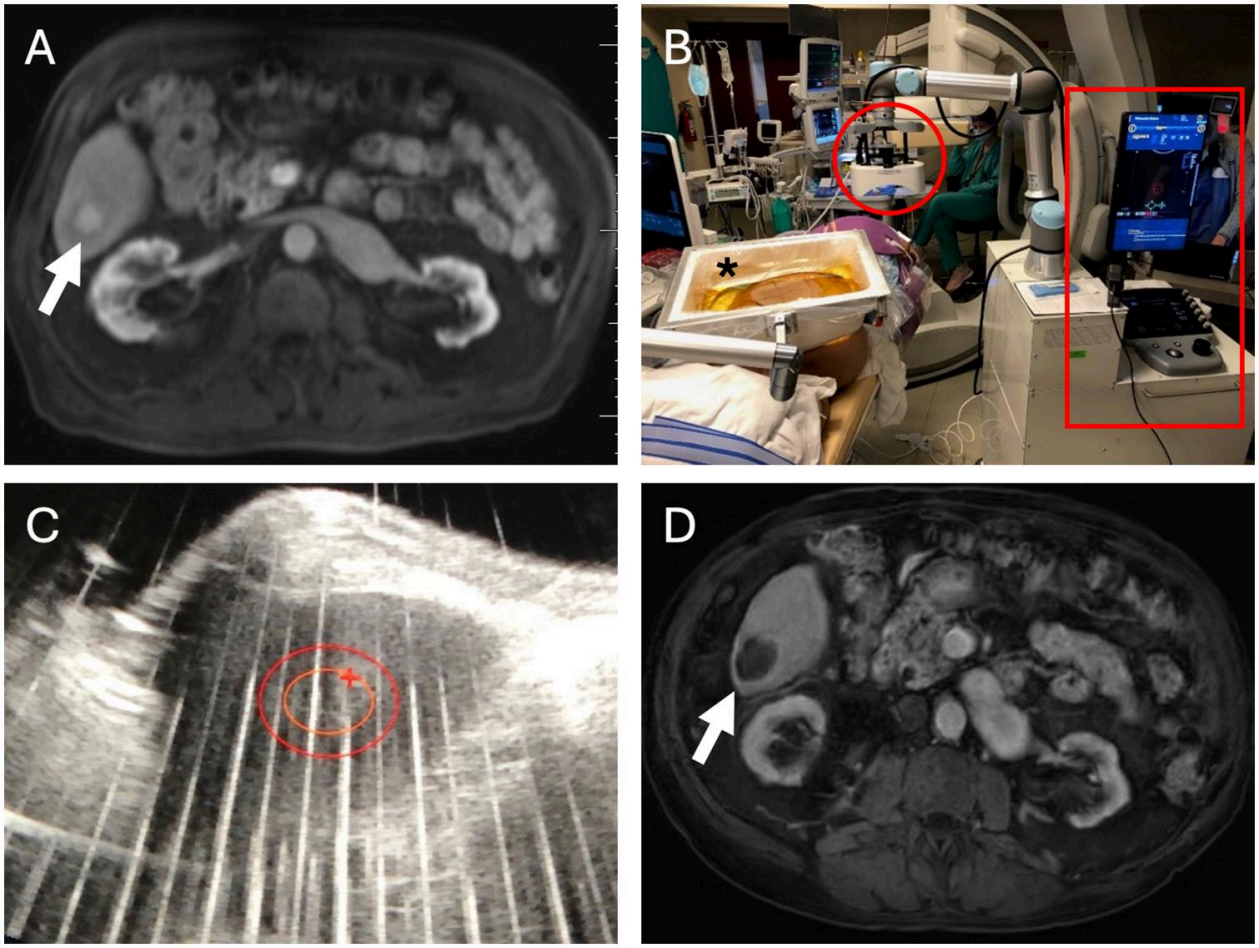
Histotripsy for primary liver cancer. 72-year-old male with alcoholic cirrhosis with 2.1 cm hepatocellular carcinoma: (A) axial contrast-enhanced MRI shows a 2.1 cm arterially enhancing lesion (arrow) in segment VI; (B) the patient was treated with the Edison System (HistoSonics, Inc.), which includes the probes (circle), computer unit (rectangle), and a water bath (*) placed over the patient’s abdomen, over which the system delivers pulsed sound energy to the hepatic tumor; (C) ultrasound imaging demonstrates the sound energy pulses during treatment of the target tumor (orange oval), centered within the total ablation zone (red oval); (D) 1-month post-treatment contrast-enhanced MRI demonstrates complete response with no enhancement in the treated area (arrow).
